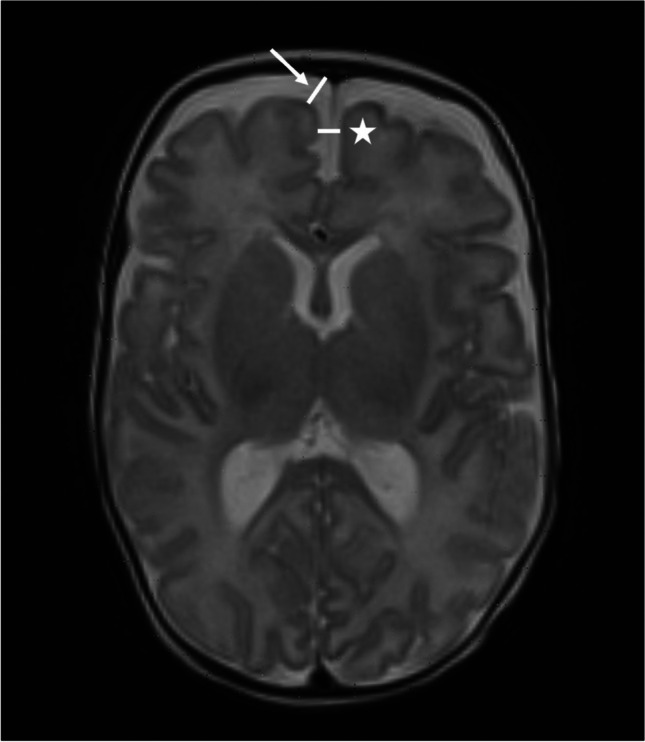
A 13-week-old girl born extremely premature at 27 gestational weeks with magnetic resonance imaging performed at term-equivalent age. Axial T2-weighted image shows measurements of sinocortical width on the right side (*arrow*) and interhemispheric distance (*asterisk*) at the level of foramen of Monro
